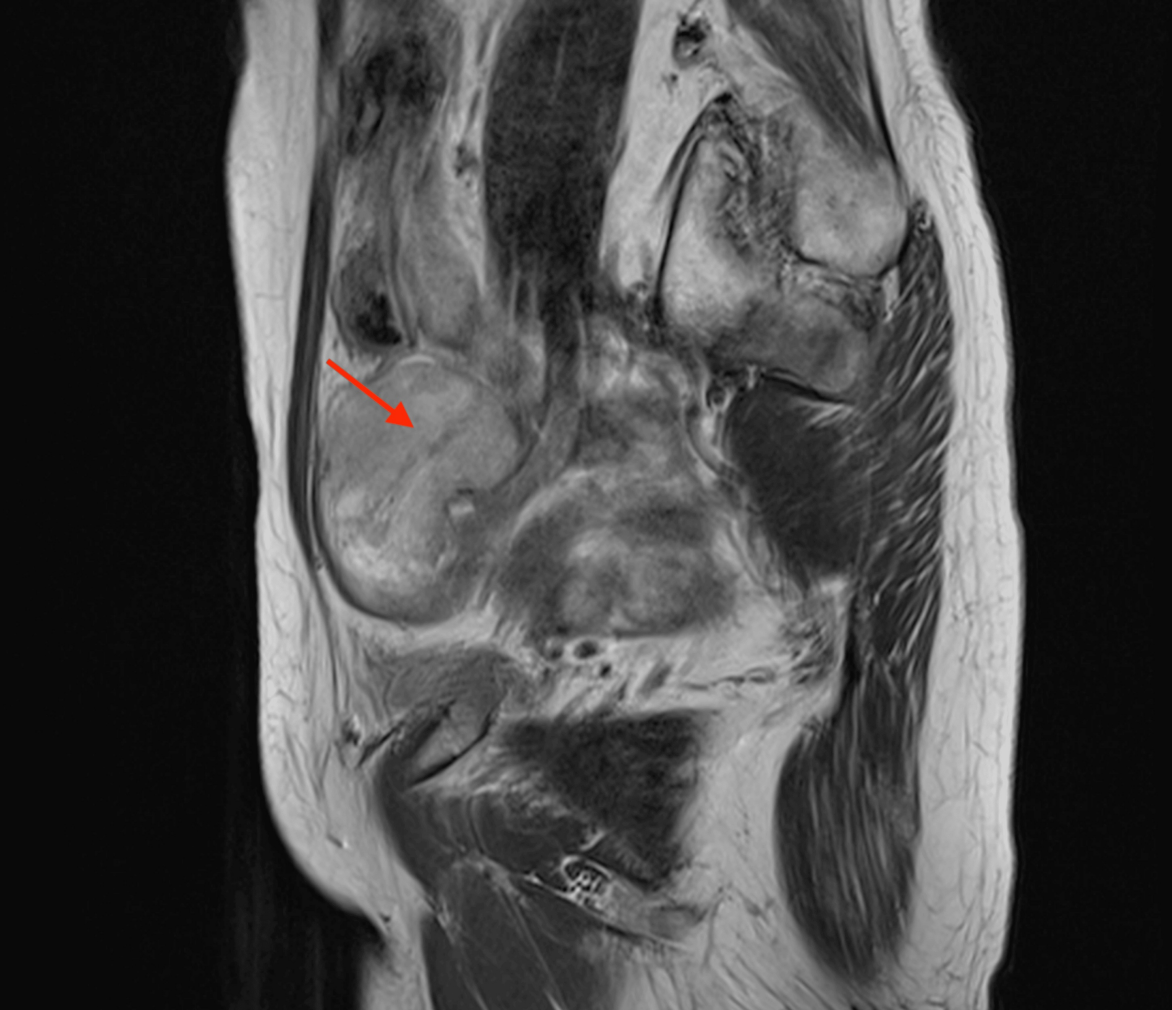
This is the same patient as shown in Figure 8. On the sagittal T2-weighted image, the lesion demonstrates heterogeneous signal intensity with alternating high- and low-signal layers, consistent with the mille-feuille sign (arrow).
